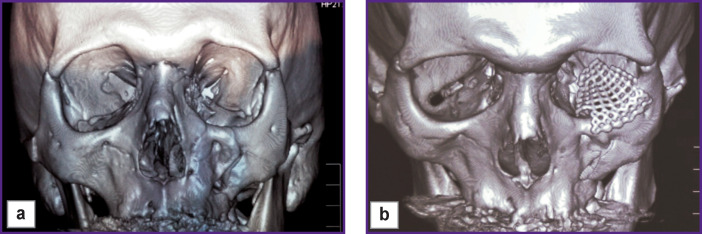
Patient K. 3D CT reconstruction of the skull: (a) before surgery; (b) after surgery (a titanium mesh implant installed on the left orbital floor and the infraorbital rim, partly on the body of the zygomatic bone)
